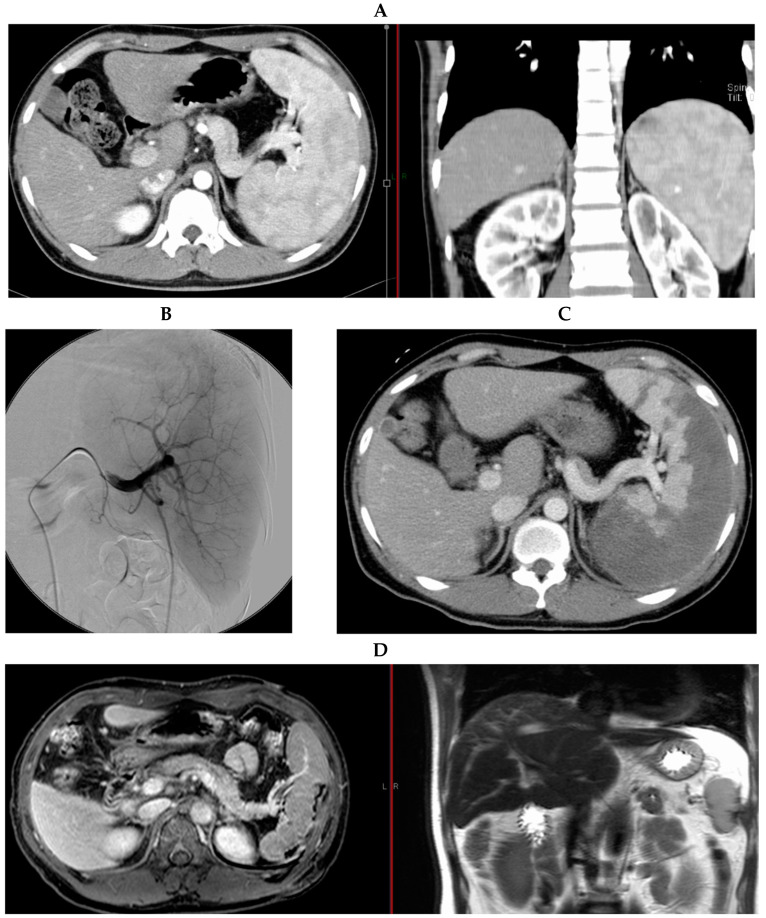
Imaging results for the patient. (A) Contrast-enhanced abdominal CT scan before PSE. The contrast-enhanced abdominal CT scan shows that the spleen is more than 12 cm in size at the coronal section, indicating splenomegaly. (B) Splenic angiography. Splenic angiography was performed to identify the embolized subregions of the spleen. (C) Spleen contrast-enhanced abdominal CT scan. The embolized areas of the spleen after PSE were confirmed with this contrast-enhanced CT scan. (D) Abdominal MRI conducted 5 years after PSE. The MRI was performed to confirm liver tumors 5 years after the PSE. There was no recurrence of splenomegaly.
